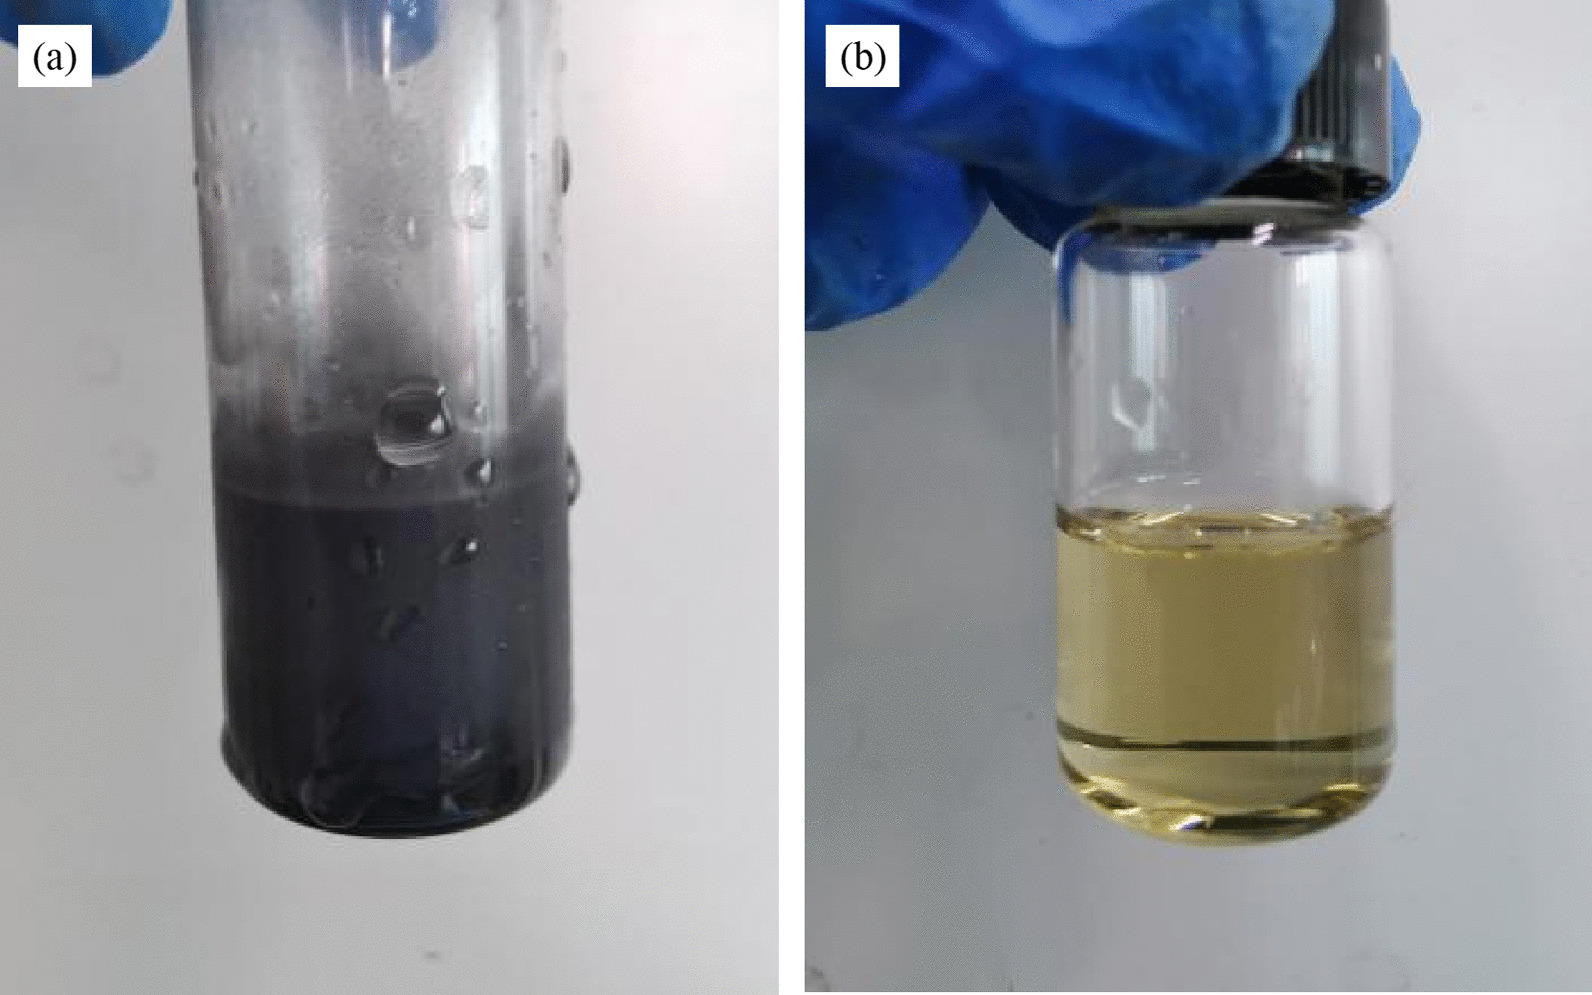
MoS_2_ nanosheet solution. a The ultrasonic MoS_2_ solution before centrifugal cleaning, b the centrifuged MoS_2_ nanosheet solution
